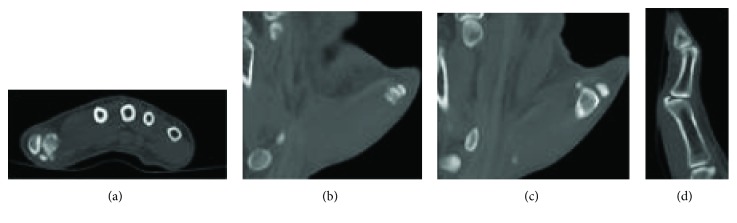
CT scan obtained three weeks after injury. Workup by primary physician included an ultrasound, which was nondiagnostic, and then this CT scan on the same day. Images demonstrated dorsal subluxation of the thumb MCP joint with incarcerated radial sesamoid.
